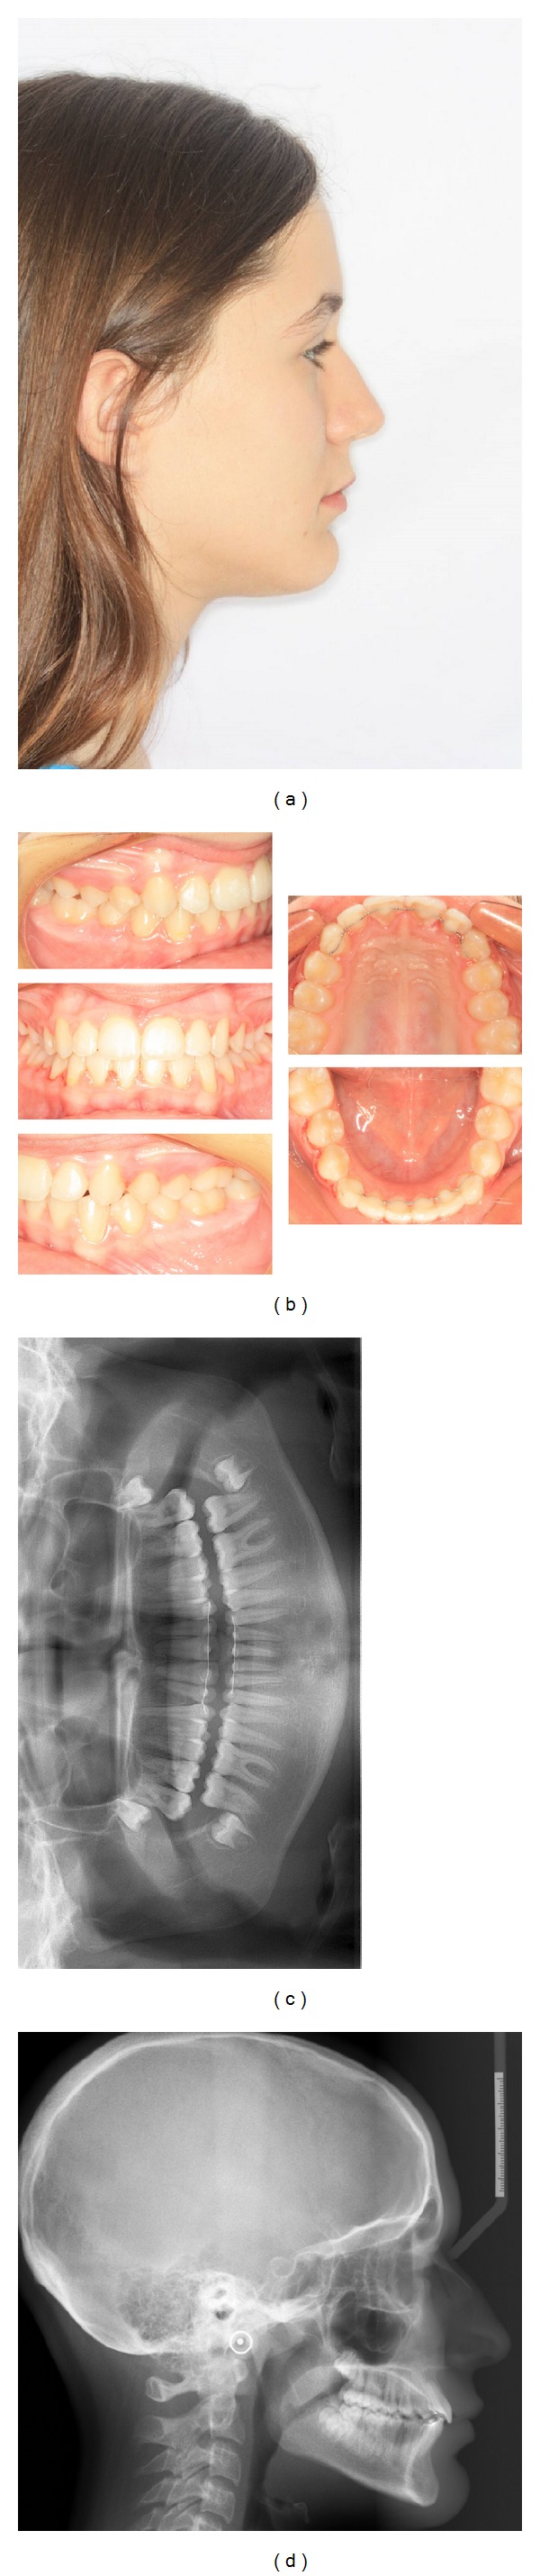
Posttreatment records: (a) extraoral profile photo, (b) dental casts, (c) panoramic radiograph, and (d) cephalometric radiograph.
